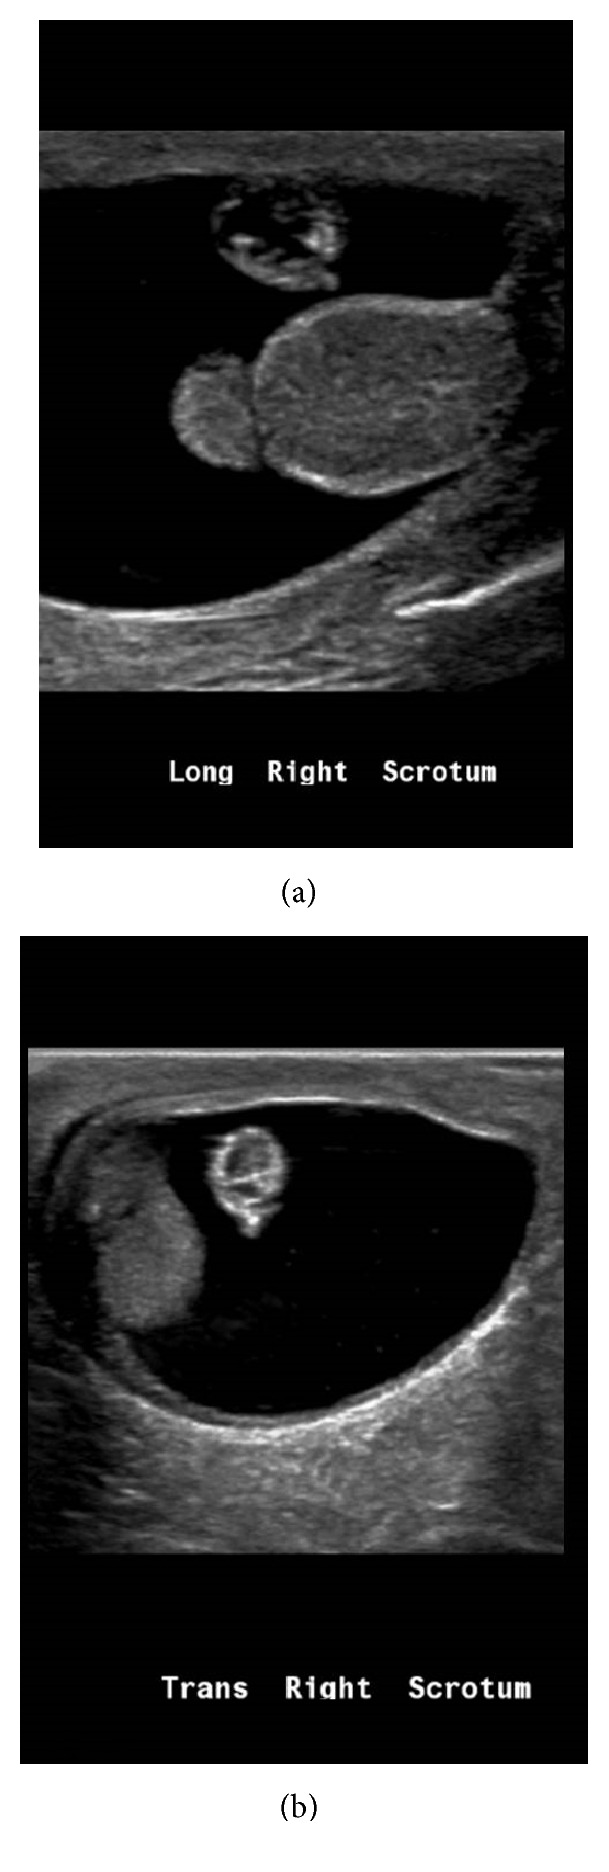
(a) Initial scrotal ultrasound showing large avascular extratesticular mass measuring 0.5 × 0.55 × 0.57 cm. (b) Six-week follow-up scrotal ultrasound shows decrease in size of extratesticular mass measuring 0.39 × 0.37 × 0.44 cm.
